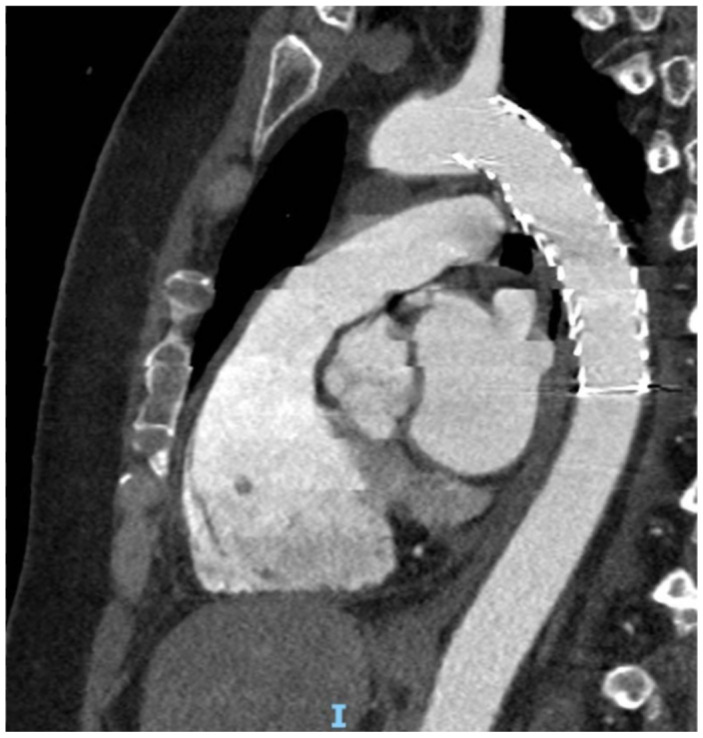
Post-operative CTA showed correct positioning and patency of the endoprosthesis (I: With complete exclusion of aortic rupture and complite obliteration of the tract).
